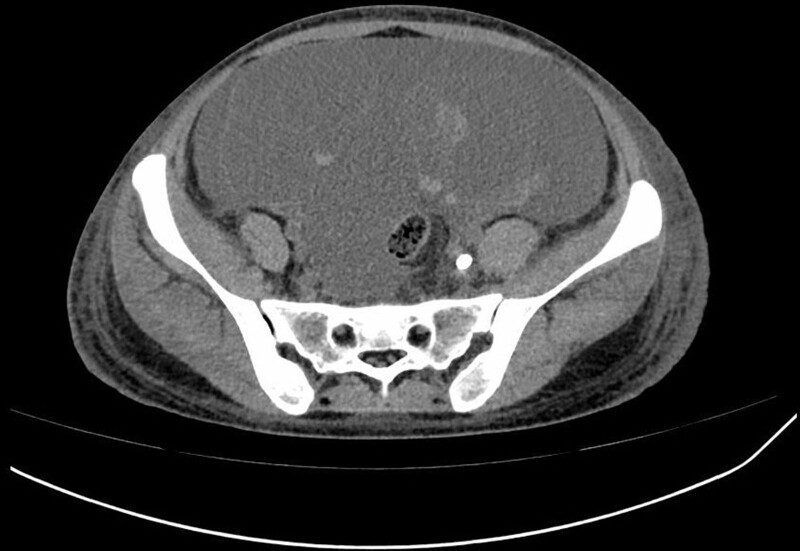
Abdominal computed tomography on the 40th day of admission showing multi-room cysts in the pelvis which were close with bilateral adnexal areas.
